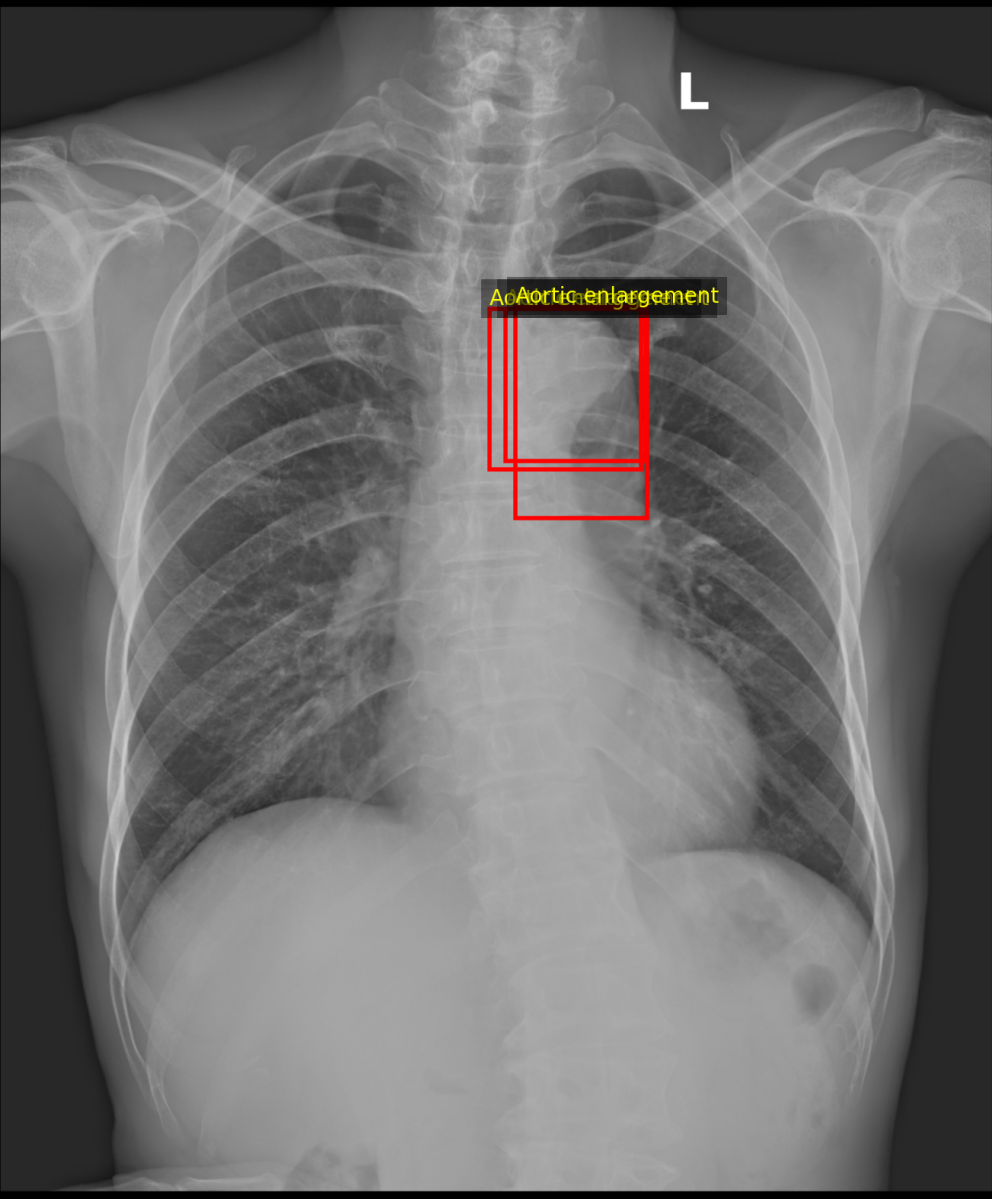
Radiologist bounding-box annotations for the same case, indicating an ectatic aortic contour along the left upper mediastinum. The red frame denotes the expert-annotated region of interest, and the letter “L” indicates the left side of the chest radiograph. The main Grad-CAM hotspot in Figure 10 overlaps this region.
